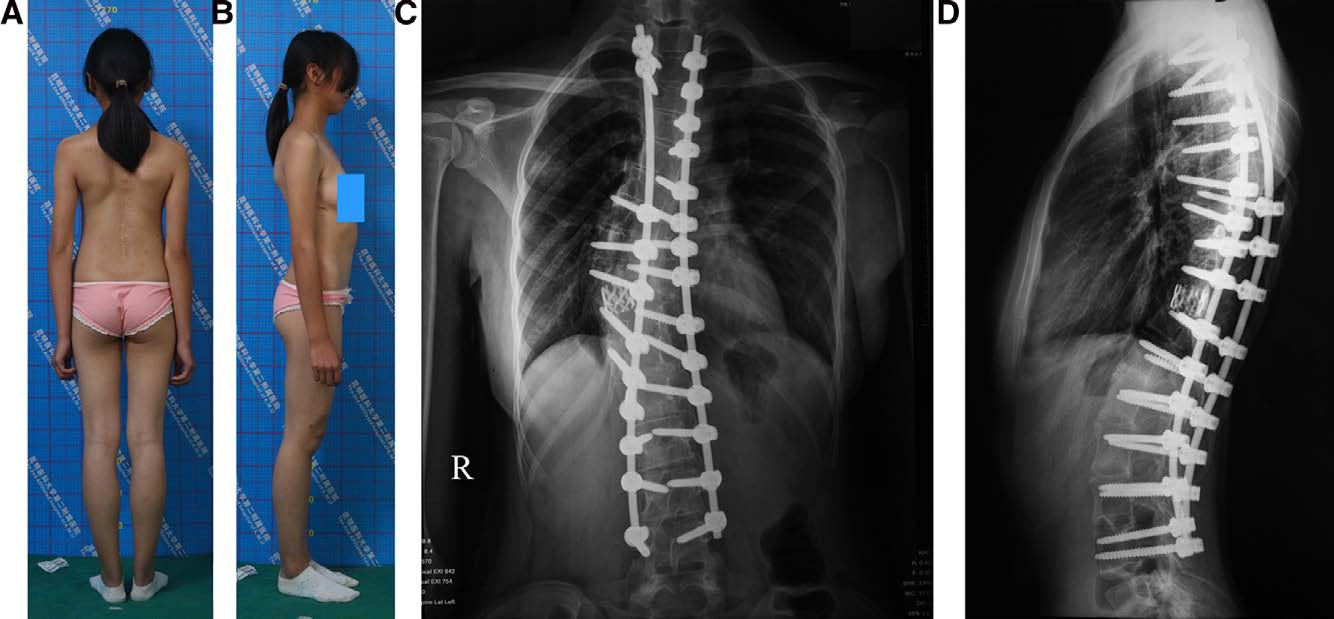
Forty-eight months after surgery. (A) Posterior view. (B) Lateral view. (C) An AP X-ray shows a Cobb angle of the coronal main curve of 14°, RSH of 0 cm, and C7-CSVL of 0.3 cm. (D) A lateral X-ray shows the Cobb angle 25° and the SVA 1 cm. AP = anteroposterior, C7-CSVL = C7-central sacral vertical line, RSH = radiographic shoulder height, SVA = sagittal vertical axis.
